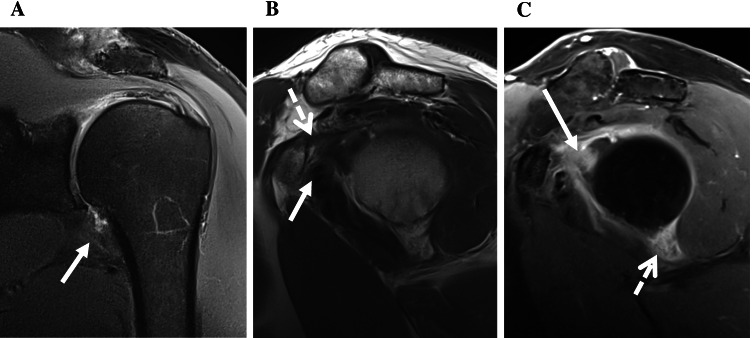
MR images from the left shoulder of a 53-year-old male with clinical signs of ACS. A Fat-saturated oblique coronal PDw image displays thickening and mild signal increase of the capsule of the axillary recess (arrow) indicating ACS. B T2w oblique sagittal image shows obliterated subcoracoid fat triangle (arrow) as well as a thickened CHL (dotted arrow) and capsule in the rotator interval. C T1w fat-saturated oblique sagittal image after IV contrast demonstrates capsular enhancement in the rotator interval (arrow) and axillary recess (dotted arrow)
